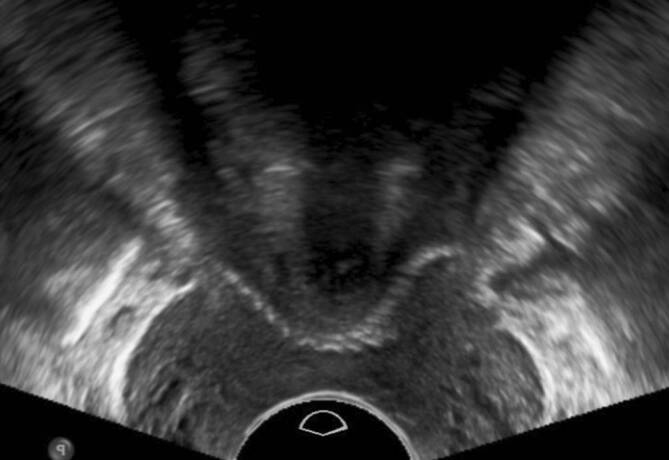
## Pelvic-Floor-Sonographie im postoperativen Komplikationsmanagement
